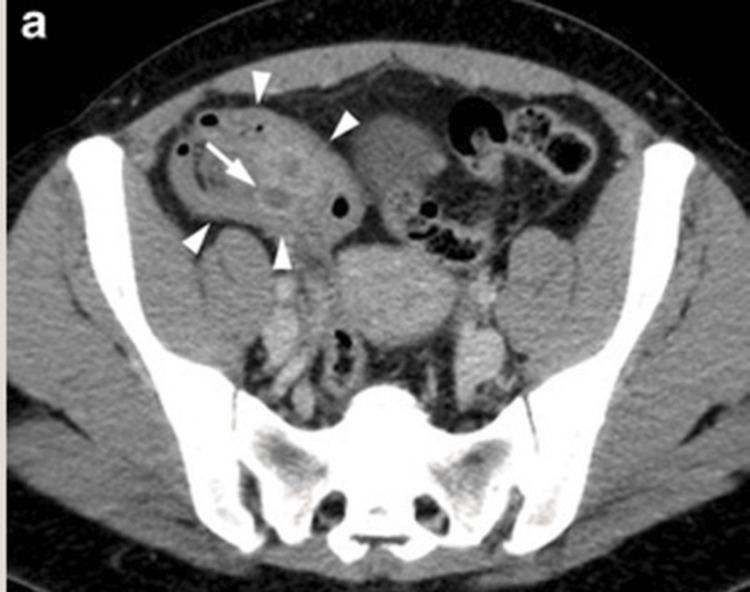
Contrast-enhanced CT showing appendicular lump (phlegmon) formation in a case of acute appendicitis.
